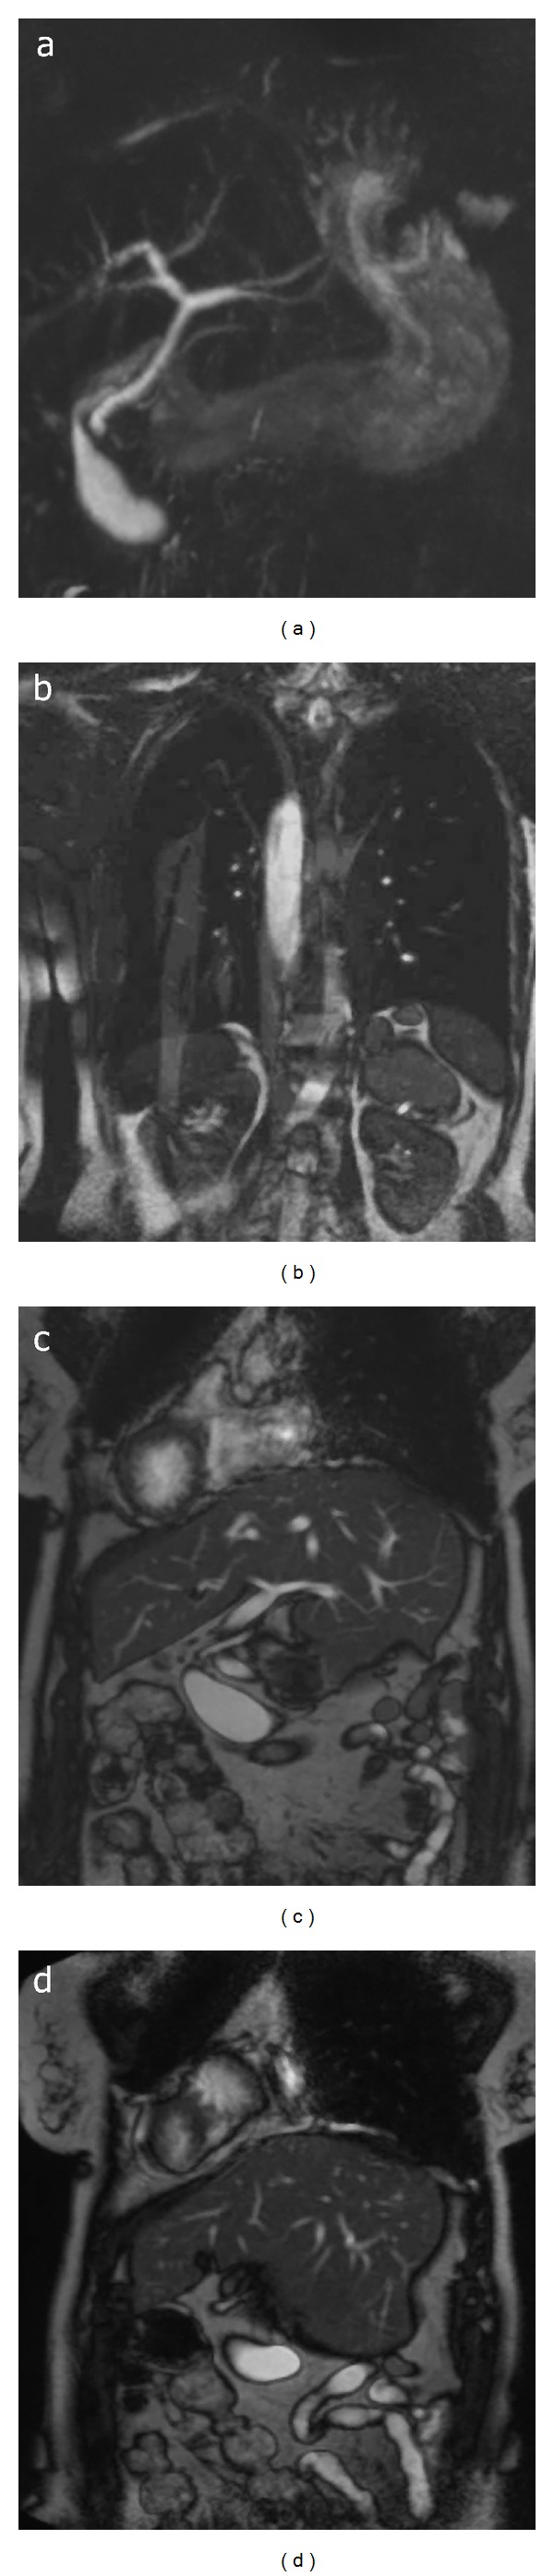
MRCP and BTFE-BH SENSE sequence on MRI. (a) MRCP imaging shows that the liver is left dominant in the midline, the common hepatic duct and common bile duct extend from the left superolateral to the inferomedial, and the pancreas is in normal location. (b) On coronal BTFE-BH SENSE sequence on MRI, the spleen is seen with three foci at the left below the liver congruent with polysplenism. (c) and (d) On coronal BTFE-BH SENSE sequence on MRI, the liver is left dominantly located at the both sides of the midline and the portal vein drains into the liver leading from the left. The intestines seem to be congruent with malrotation, and, predominantly, large intestines on the right-hand side and small intestines on the left-hand side according to the midline.
